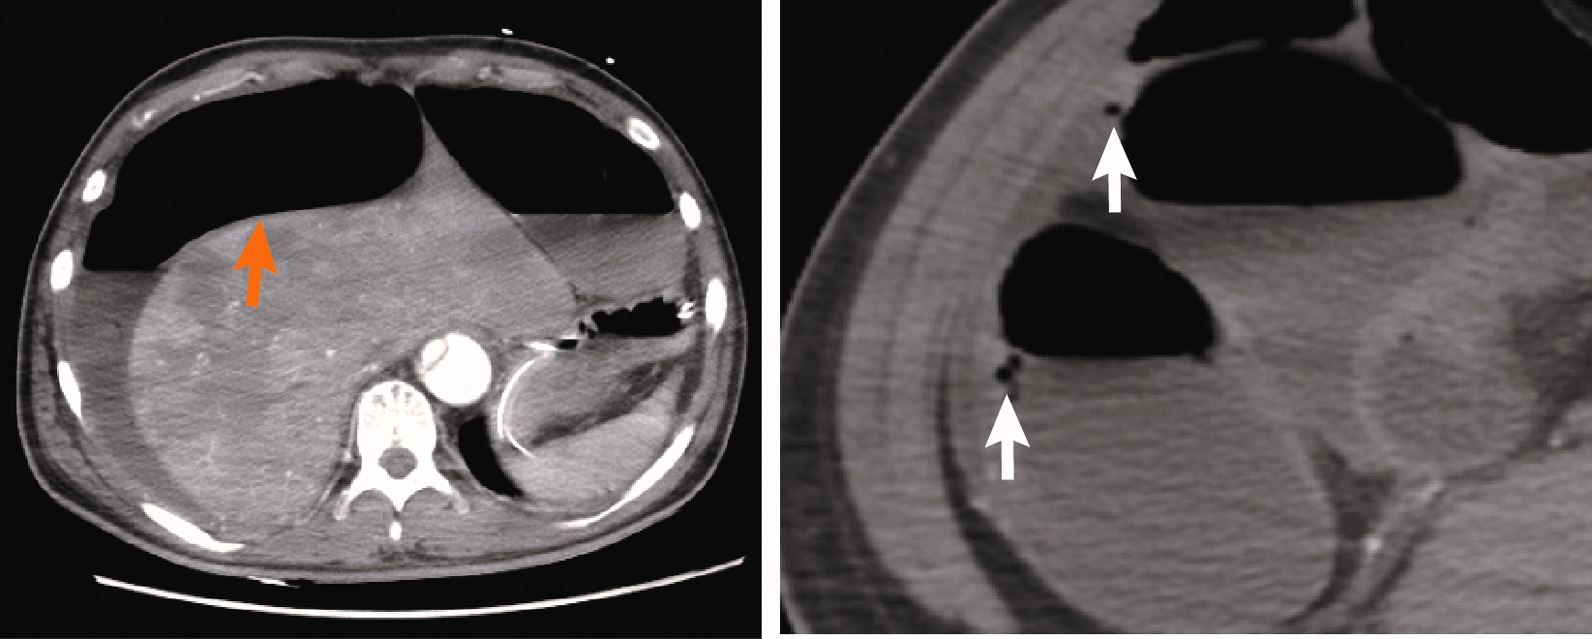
Abdominal computed tomography of the patient revealed a distended bowel with a paper-like thin wall (orange arrows) and gas accumulation (white arrows) in the small intestine wall
